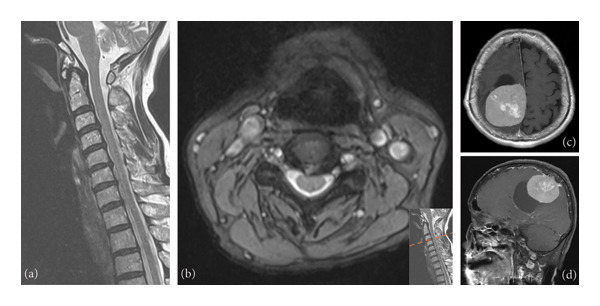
Case 3: Images of cervical magnetic resonance imaging. (a) Sagittal view showing disc bulging at C3‐4, C4‐5, and C5‐6 with mild cord compression. (b) Axial views of C3‐4. (c) Sagittal view of brain MRI. (d) Axial view of brain MRI revealed a sizable 7.0‐cm lesion characterized by cystic formation in the right parietal region. This lesion was causing compression of the cortex surrounding the right central sulcus, accompanied by edema extending into the subcortical white matter.
